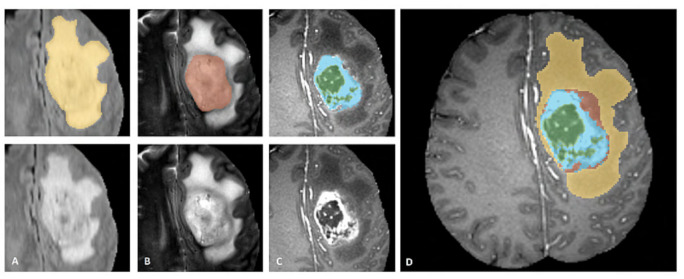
BraTS task description [36]. The whole tumor is visible in FLAIR (A), the tumor core in T2 (B), the enhancing tumor structures in T1c (blue), surrounding the cystic/necrotic components of the core (green) (C). Combined segmentation give the final labeled image (D): edema (yellow), nonenhancing solid core (red), necrotic/cystic core (green), enhancing core (blue).
